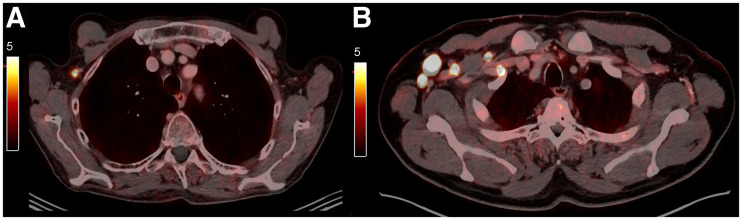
Representative PSMA PET/CT images of a patient with VAL (A) and of another patient with axillary lymph node metastasis (B).
